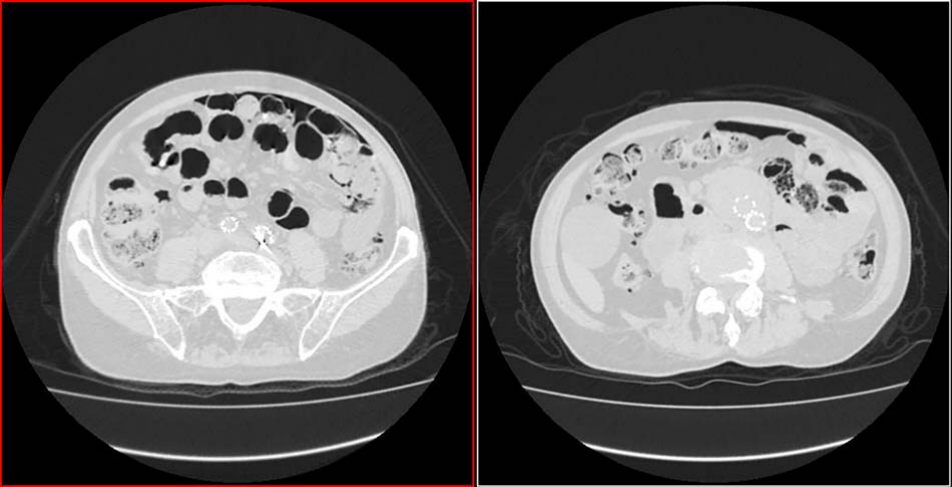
Coronal CT image in lung window showing pneumoperitoneum and ‘bubbles’ within the walls of small intestine and in the mesentery.
